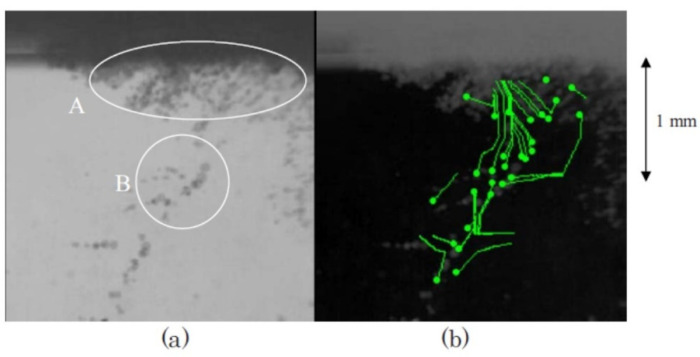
Cavitation bubbles under an ultrasonic horn at 29 kHz and 5 W observed by a high-speed video camera [19]. The frame (b) is the result of the analysis of the bubble motion. The small circles are the starting points for the analysis and the curves are the calculated streamlines of bubbles. In the frame (a), the bubble clouds A and B are marked by circles. Copyright 2008, with the permission from American Physical Society.
